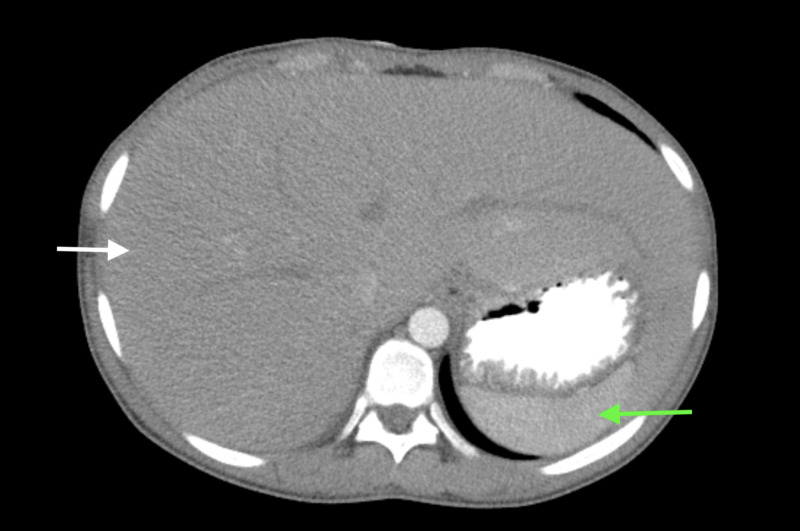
Computed tomography (CT) of the abdomen A transverse view of abdominal CT scan showing massive hepatomegaly (white arrow) with mild diffuse nonspecific hepatic steatosis as indicated with the difference in intensity between liver (white arrow) and spleen (green arrow).
